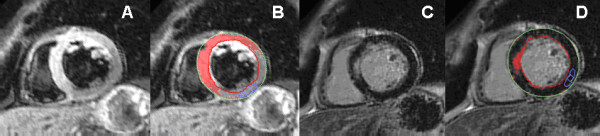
Assessment of myocardial salvage after acute, reperfused myocardial infarction. A: T2-weighted CMR showing high signal intensity of the anterior, anteroseptal and inferoseptal segments (area at risk). B: Computer-aided signal intensity analysis of the T2-weighted image normalized to normal, uninjured myocardium. Red indicates a signal intensity of >2 standard deviations above remote, uninjured myocardium. C: Contrast-enhanced image (late gadolinium enhancement) showing high signal intensity reflecting increased contrast accumulation in necrotic myocardium. D: Computer-aided signal intensity analysis of the late gadolinium enhancement image with color-coded display of relative signal intensity, normalized to remote myocardium. Red indicates a signal intensity of >5 standard deviations above remote, uninjured myocardium. The comparison of edema (panels A, B) with necrosis (panels C, D) shows myocardial edema in areas without necrosis, indicating major myocardial salvage.
